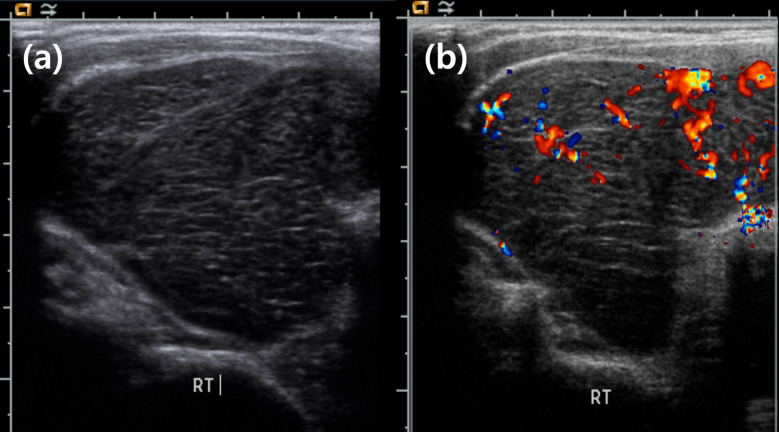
Ultrasound appearance of thyroid lymphoma. (A) Large solid mass with very hypoechoic feature. (B) Increased vascularity in mass.
